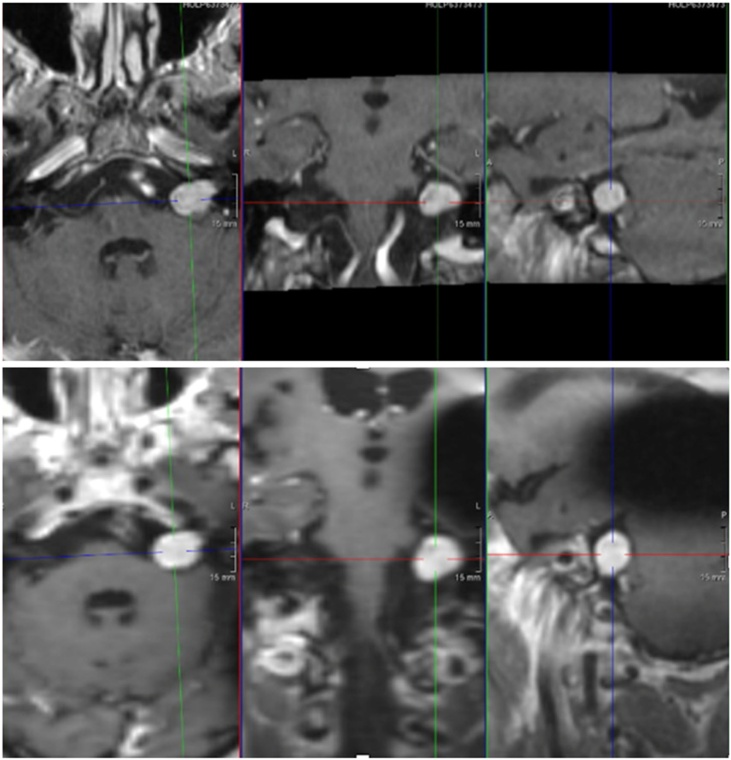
Cochlear implantation in a patient with bilateral hearing loss and a stable left VS. A 69-year-old received a left CI with no tumor removal. Above, MRI performed before cochlear implantation shows a 14 mm VS. Below, MRI performed following cochlear implantation. Note there is a black artifact which does not interfere with the correct vision of the tumor in the 3 planes (axial, coronal and sagittal).
